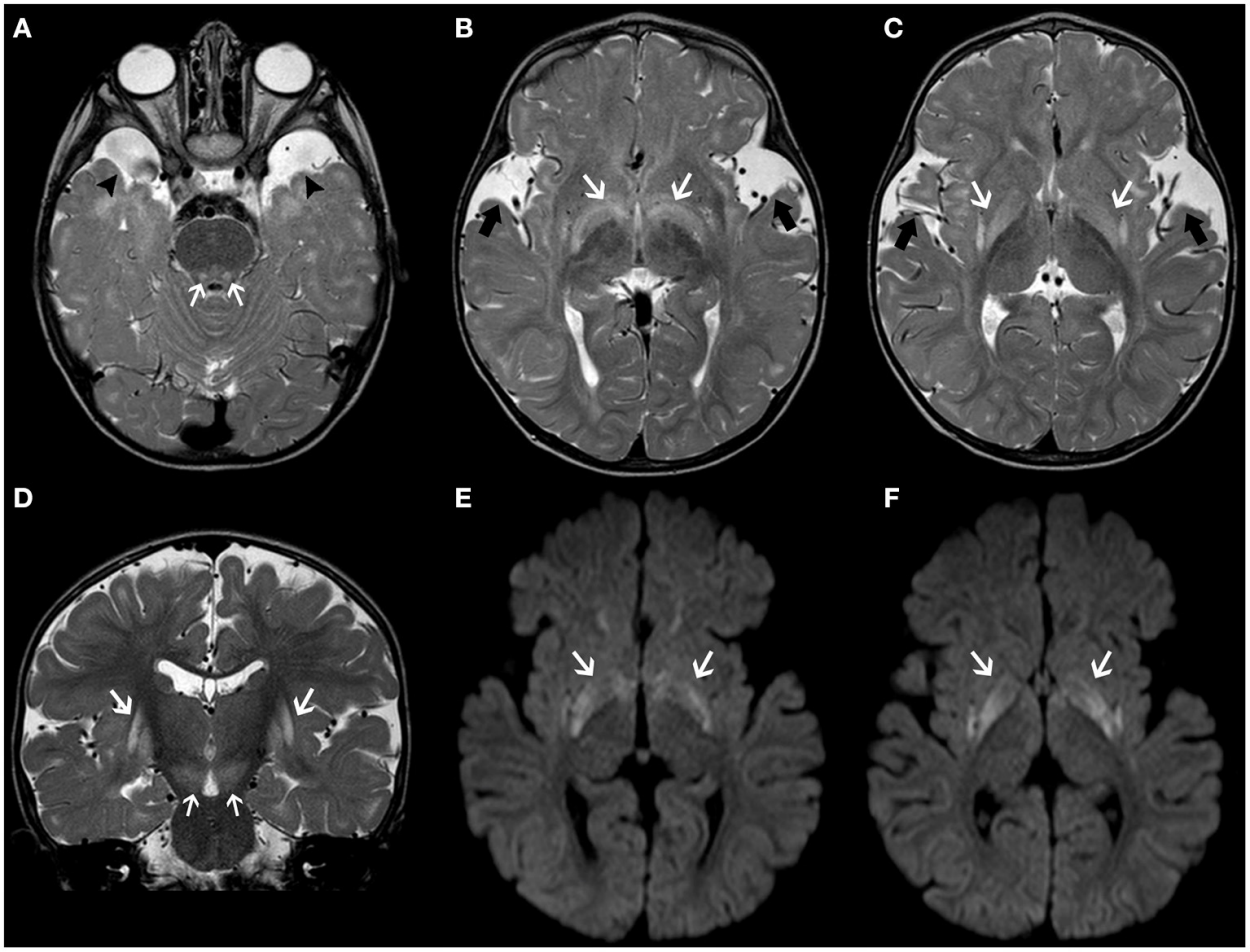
Longitudinal brain MRI findings in Glutaric Aciduria type I. Axial (A–C) and coronal (D) T2TWI of an affected 1.3-years-old boy demonstrates enlargement of the anterior temporal subarachnoid spaces (black arrowheads) and widening of the Sylvian fissures (thick black arrows) due to incomplete opercularization. There are also bilateral, symmetric hyperintensities in the globus pallidus/posterior putamen, substantia nigra and central tegmental tracts (white arrows). Axial b1000 images (E,F) also depict hyperintensity in the globus pallidus and posterior putamen bilaterally (white arrows). There was corresponding mild hypointensity in the ADC maps (not shown), in keeping with restricted diffusion.
